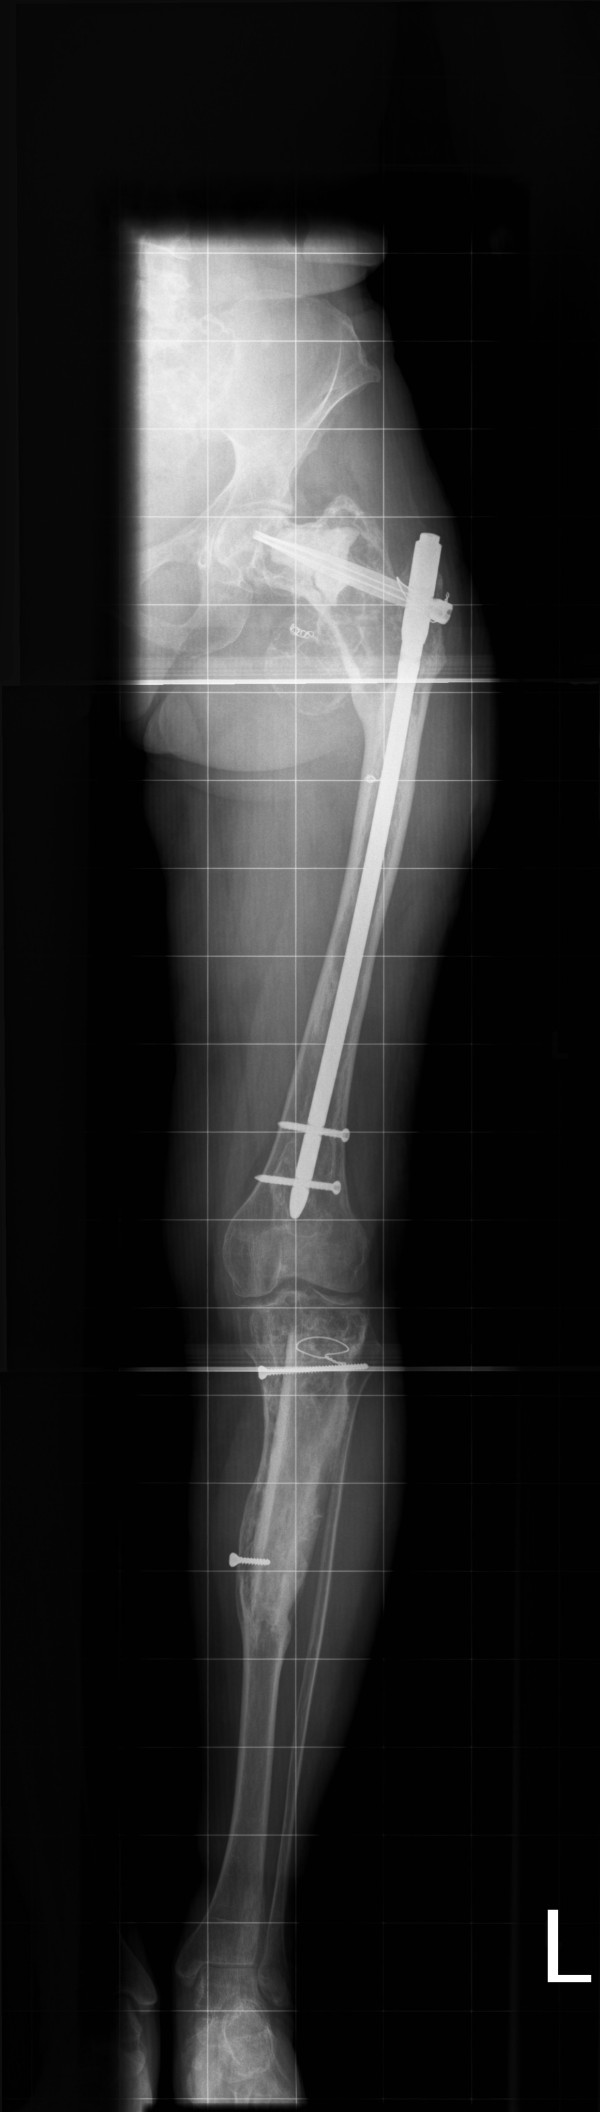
Anteroposterior X-ray of the whole left leg. Situation after fibrous dysplasia excision of the left proximal femur and left tibia with correctional osteotomy of the left hip and fixation with a proximal femoral nail, as well as allograft fixation with AO screws of the tibia.
